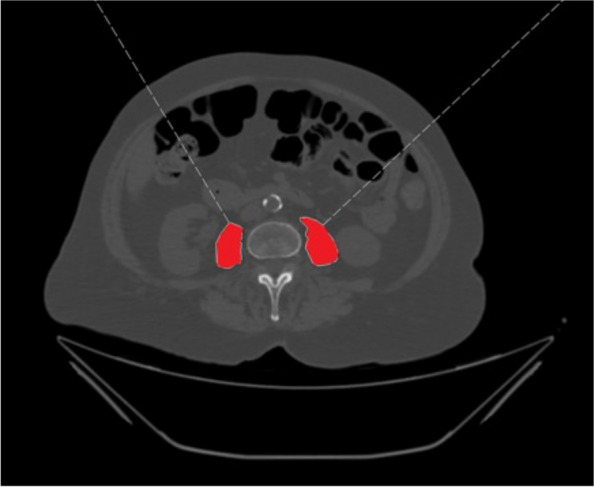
Exemplary measurement method of the psoas muscle area on an axial CT scan, the psoas muscle circumference is traced manually and the area within the tracing calculated in (cm.^2^)
